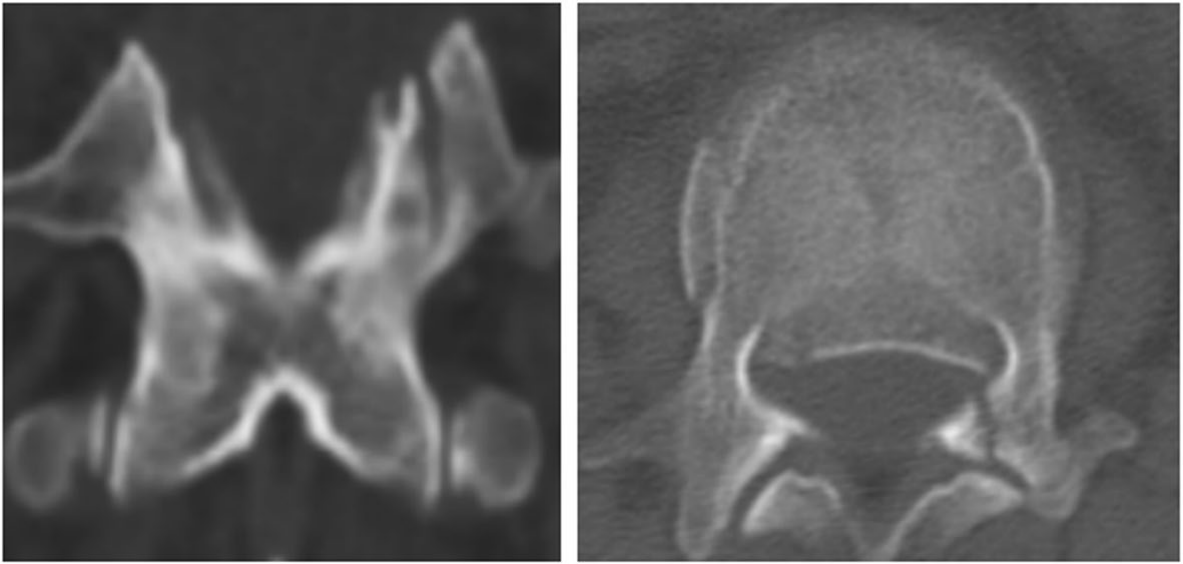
Partial and part-length laminar fractures. Reconstructed coronal computed tomographic scan revealing part-length laminar fracture. The axial plane shows complete or incomplete laminar fracture
